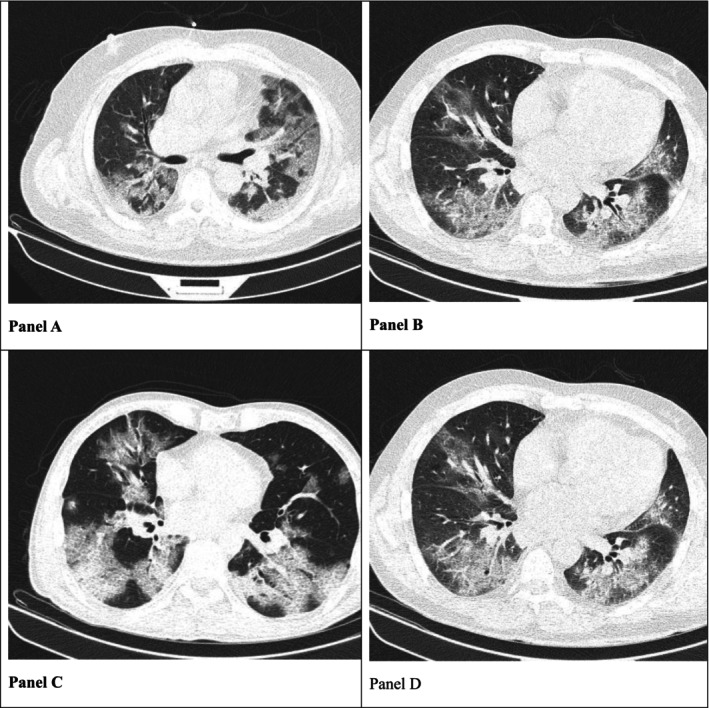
The CT images of four different patients with pulmonary involvements. Descriptions of panels: (A) non‐vaccination; (B) indeterminate vaccination; (C) partial vaccination; (D) complete vaccination. All of the four images show mainly ground‐glass opacities with peripheral and peribronchovascular distributions.
